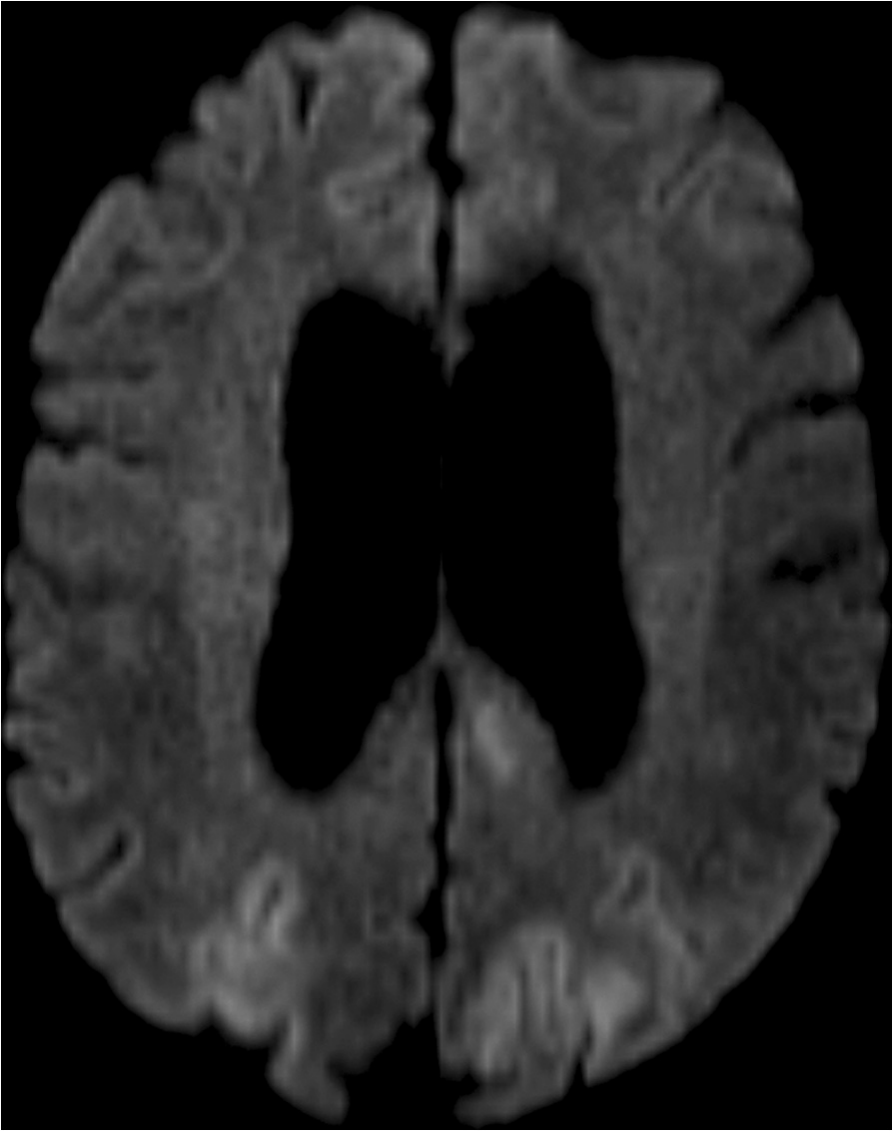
Brain axial diffusion-weighted magnetic resonance image with B1000 shows hyperintensity in the bioccipital region.
